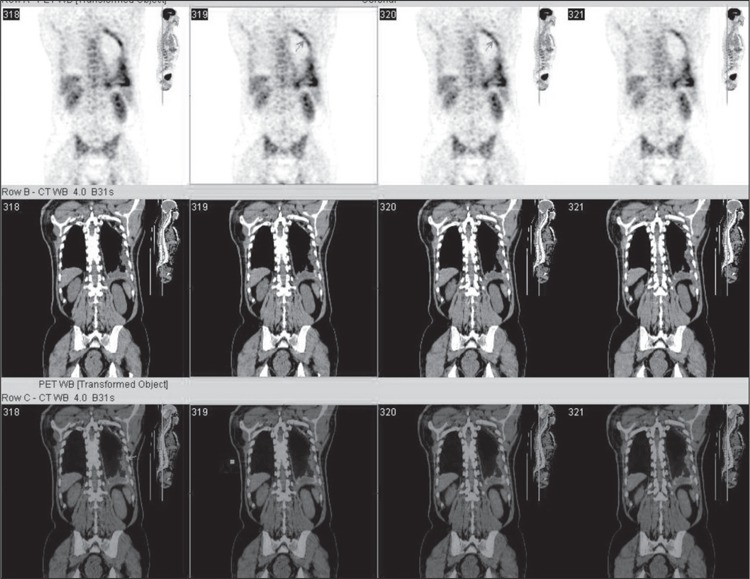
The patient wasimaged using an integratedPET/CT camera (1hour afterthe administration of 465 MBqFDG), which is consists of a6-slice CT gantry integratedon a LSO based full ring PETscanner (Siemens Biograph 6,IL, Chicago, USA). MIP PET,CT and fusion PET/CT imagesshow a diffuse F-18 FDG uptakewith a maximum standarduptake value (SUVmax) of4.2 on thickened mediastinal,costal and diaphragmatic pleurain left hemithotax indicatingpleural involvement. There isno additional focus suggestinglymphomatous disease.
